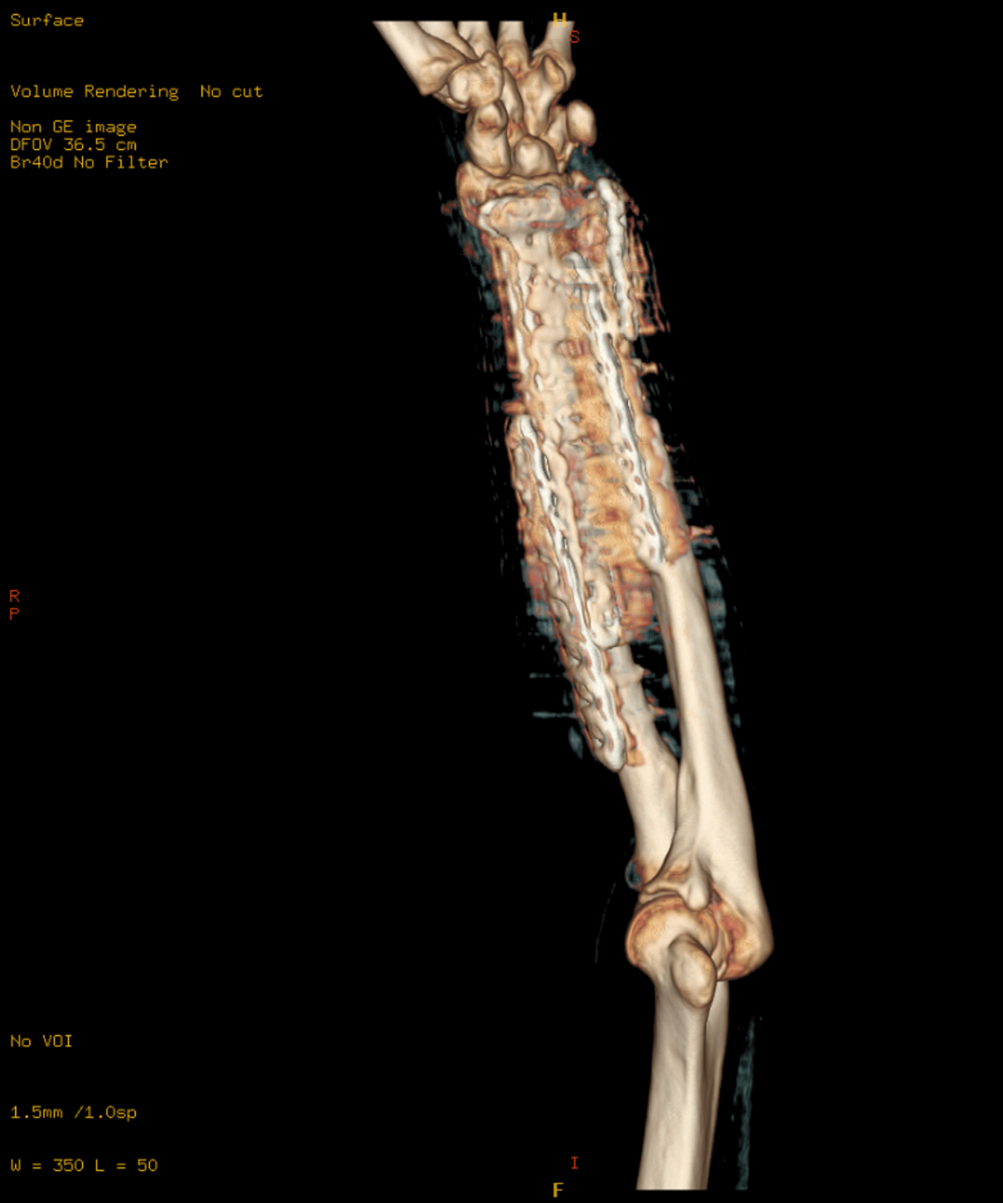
Computed tomography (CT) scan with three-dimensional (3D) reconstruction of the left forearm showing a healed fracture with a stable implant at the one-year follow-up.
